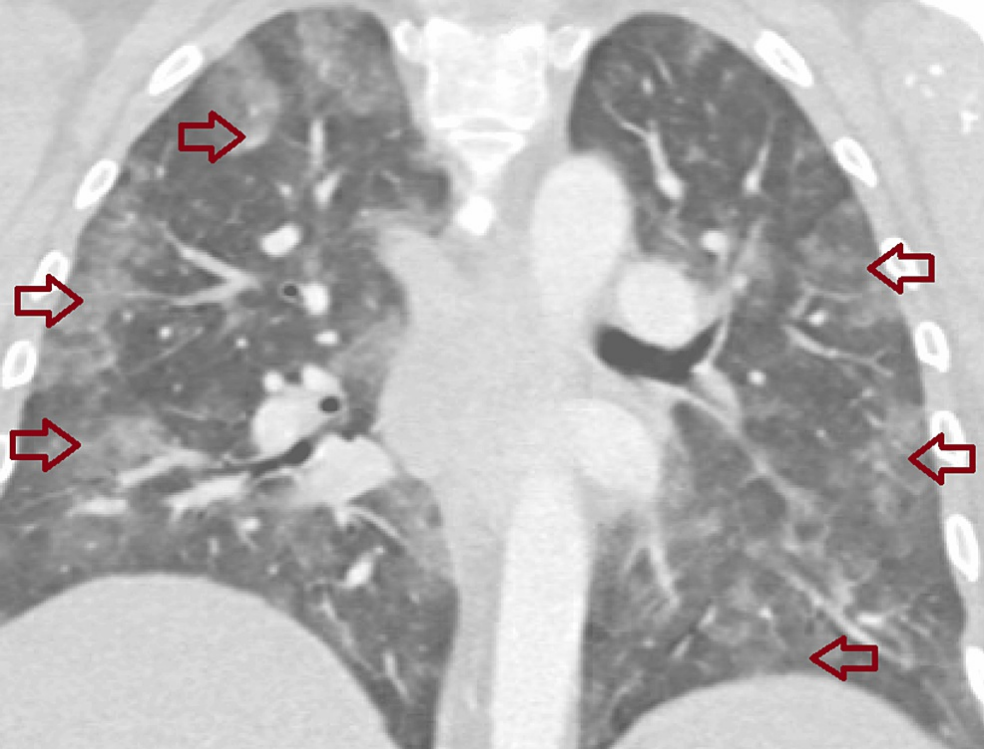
CT of the chest demonstrating multiple patchy bilateral peripheral and basilar ground-glass opacities (red arrows).
